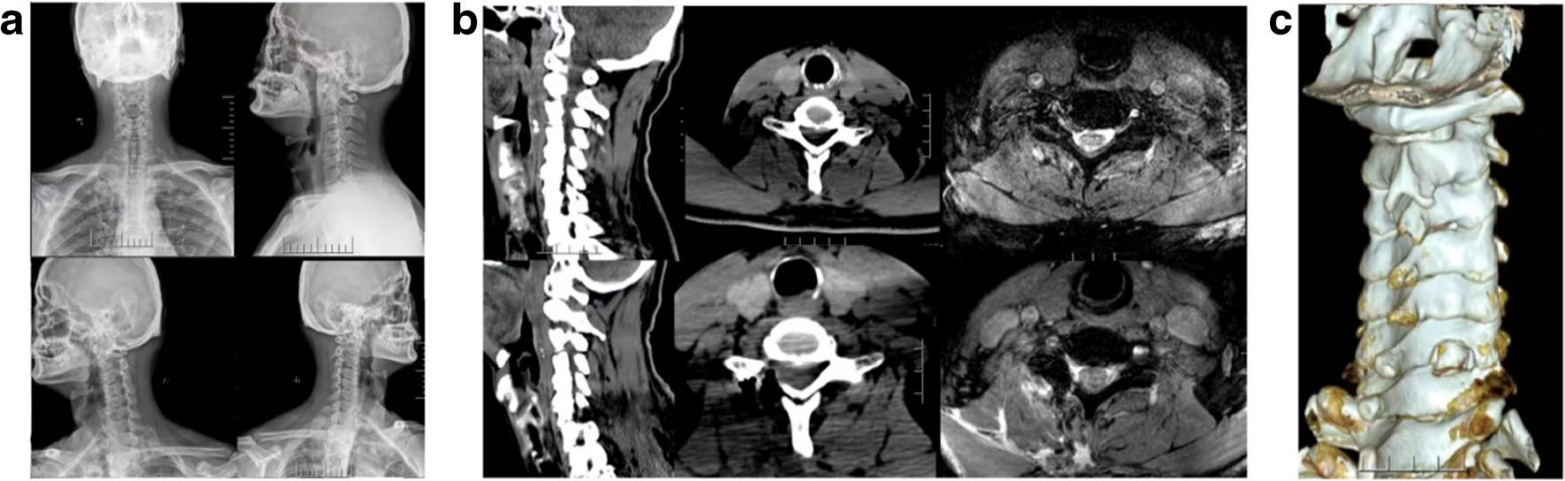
A 47-year-old male patient with right-sided neck pain accompanied by numbness in the upper limb. The surgical segment involved was C6/7. Following surgery, the patient experienced complete alleviation of symptoms. a The patient’s cervical spine X-ray in both the lateral and oblique views. b A comparison of the patient’s preoperative and postoperative CT and MRI images, with the upper portion representing the preoperative images and the lower portion representing the postoperative images. c A postoperative three-dimensional reconstruction of the patient’s cervical spine CT scan
